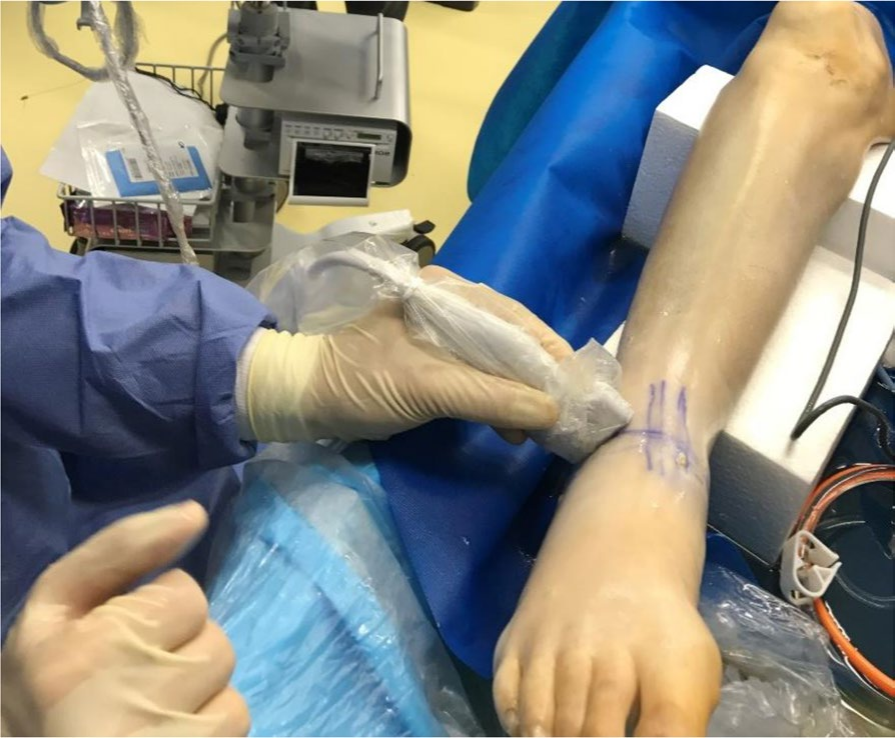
Placement of the ultrasound probe in parallel with the ligament running just above the AITFL, 30 degrees from the ankle joint
